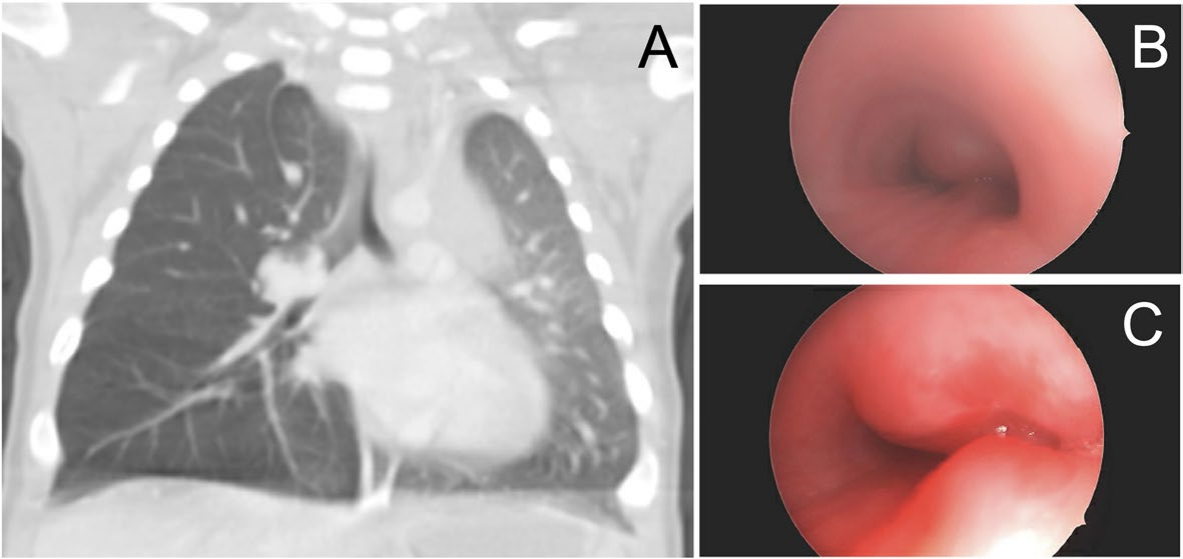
Lower airway infantile hemangioma as demonstrated by (A) chest CT showing mass compressing lumen of right mainstem bronchus, (B) bronchoscopy of proximal right mainstem bronchus on day of intubation demonstrating complete occlusion, and (C) bronchoscopy of proximal right mainstem bronchus after 6 days of treatment, demonstrating 70% occlusion
